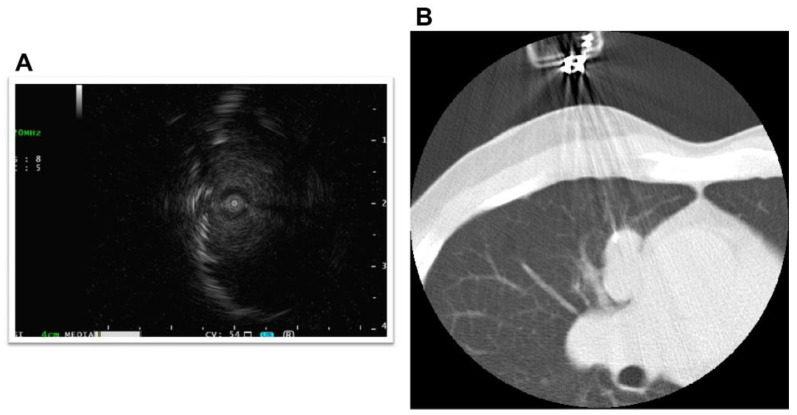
Bronchoscopy and computed tomography-guided biopsy. A thin bronchoscope, endobronchial ultrasonography, and a guide sheath were used during the bronchoscopy (A). Biopsy was performed under computed tomography guidance (B).
